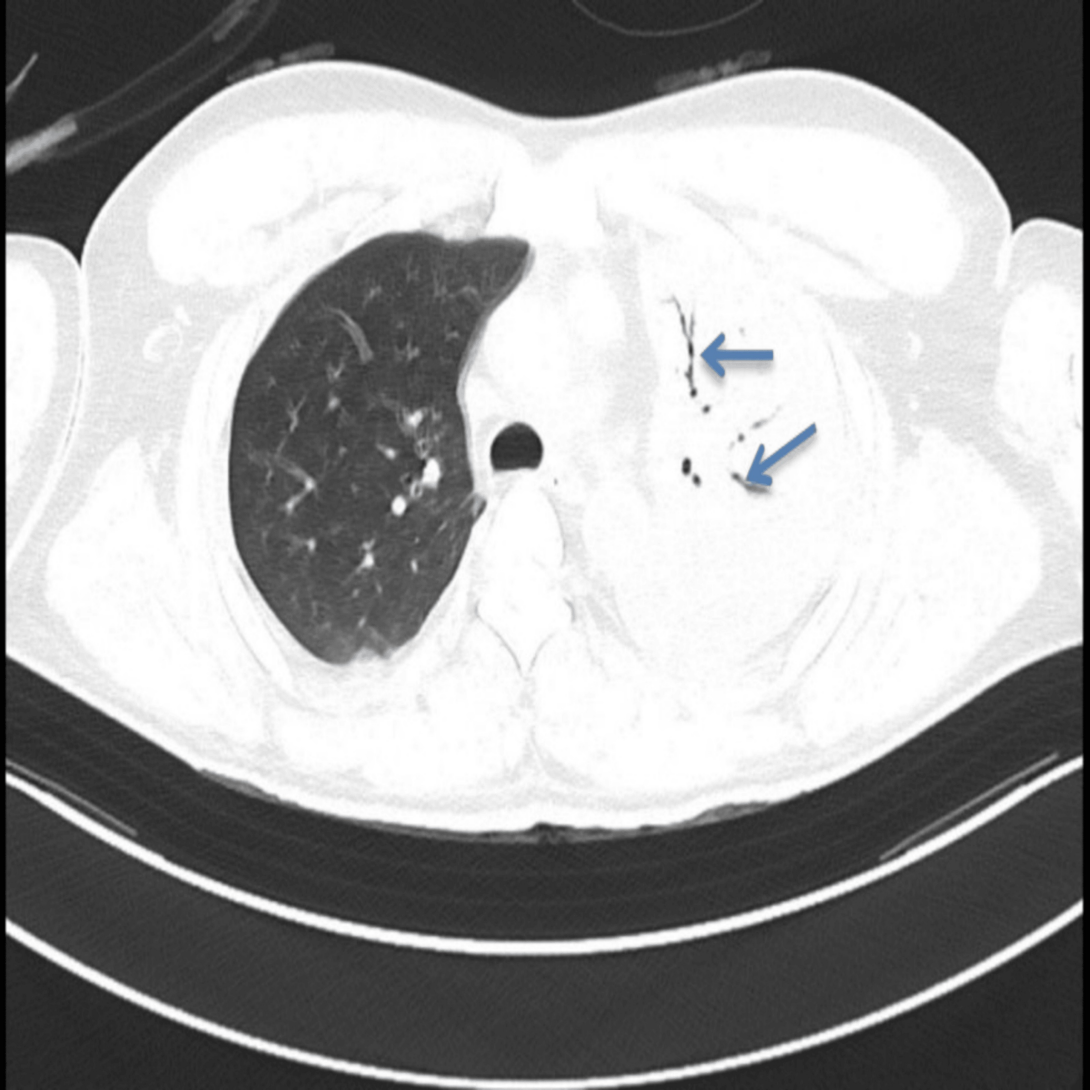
Contrast-enhanced CT thorax image (axial view) showing dense consolidation in the left lung parenchyma with air bronchogram (arrows)
